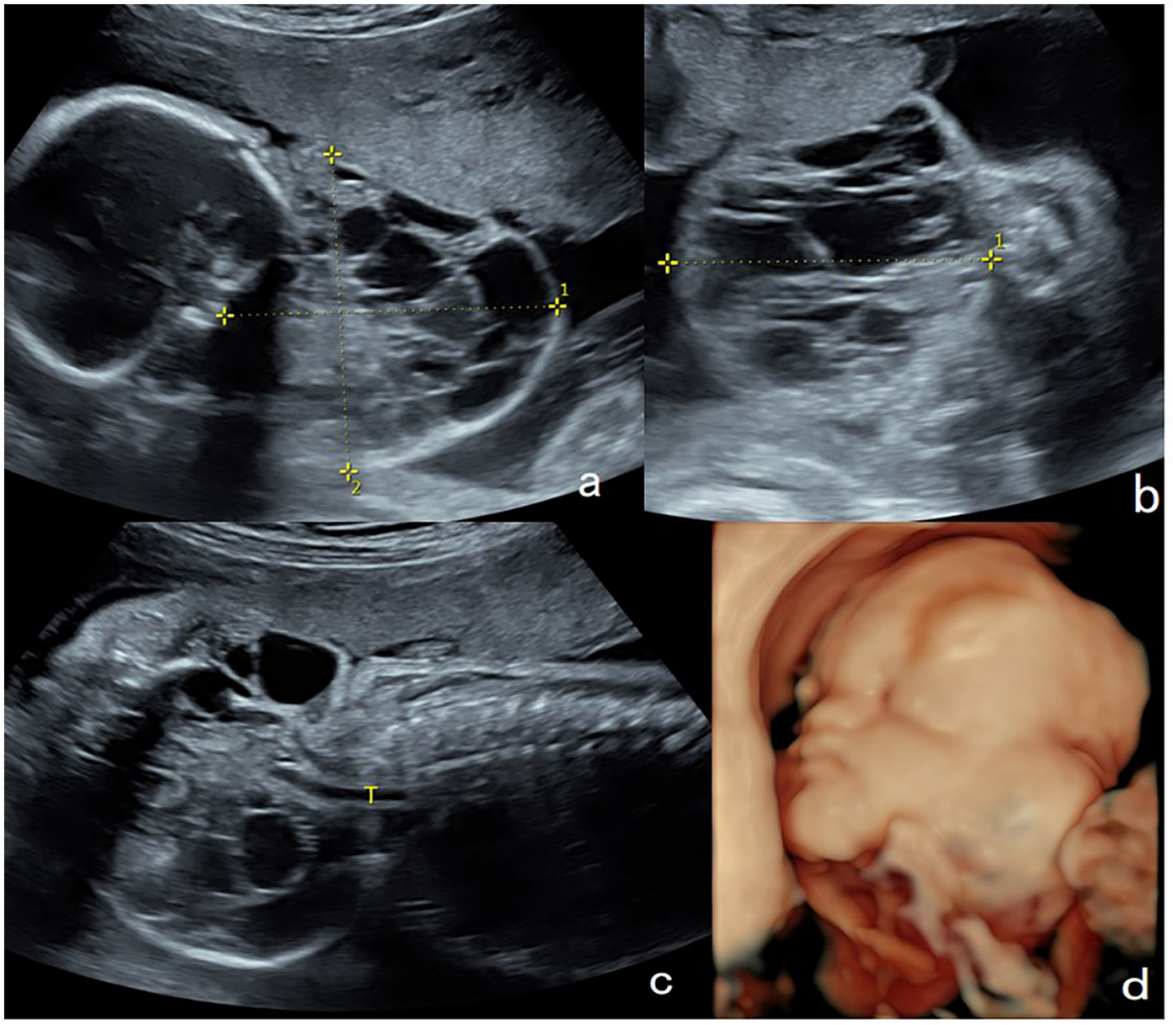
Lymphangioma in the neck of a fetus (case 9). A solid cystic mass (7.5 cm × 6.5 cm × 7.1 cm) with internal separations was found in the lateral neck of the fetus at 26 weeks of gestation. (a,b) On color Doppler flow imaging, the blood flow signals in the mass were observed in the spot strips. (c) The lower tracheal segment was visible, while the upper tracheal segment was not satisfactorily visualized. (d) Three-dimensional ultrasound showed that the mass was mainly located on the lateral aspect of the neck. The mass was 10.9 cm × 10.4 cm × 8.5 cm at 36 weeks of gestation. Elective cesarean section was performed at 36 weeks + 4 days of gestation, and tracheal intubation was successful during ex utero intrapartum treatment. The mass was surgically removed 46 days after birth and was pathologically diagnosed as a lymphangioma with a favorable outcome.
